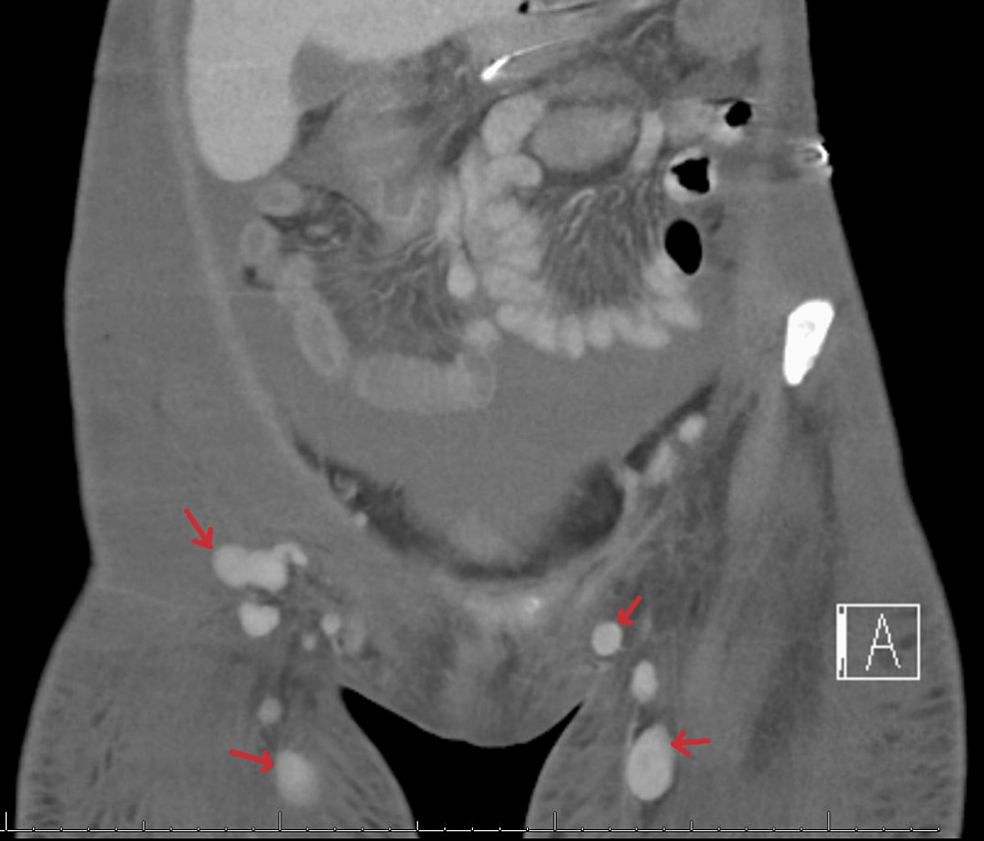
Computed tomography of the abdomen (coronal view) demonstrating bilateral inguinal lymphadenopathy (red arrows).
